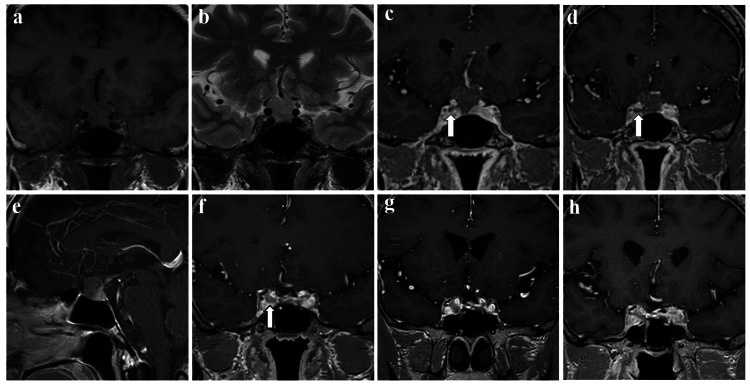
Gradual changes on magnetic resonance imaging (a, b) At the initial visit, coronal images showed a solid tumor extending into the suprasellar region (a: T1-weighted, b: T2-weighted); (c, d, e) T1 contrast images showed an extra-pituitary origin of the tumor with the normal pituitary gland and the stalk situated caudally to the left (c, d: coronal, e: sagittal). The contrast effect of the tumor was weak. Tumor invasion into the right cavernous sinus was suspected (arrow in c and d); (f, g) Postoperative T1 contrast coronal images revealed a residual tumor in the right cavernous sinus (arrow in f), which increased in size (f: four months: maximum tumor size was 4 mm, g: 10 months: maximum tumor size was 7 mm); (h) Coronal T1 contrast images taken two years after Gamma Knife stereotactic radiosurgery showed no further tumor growth (The maximum tumor size was 6 mm).
